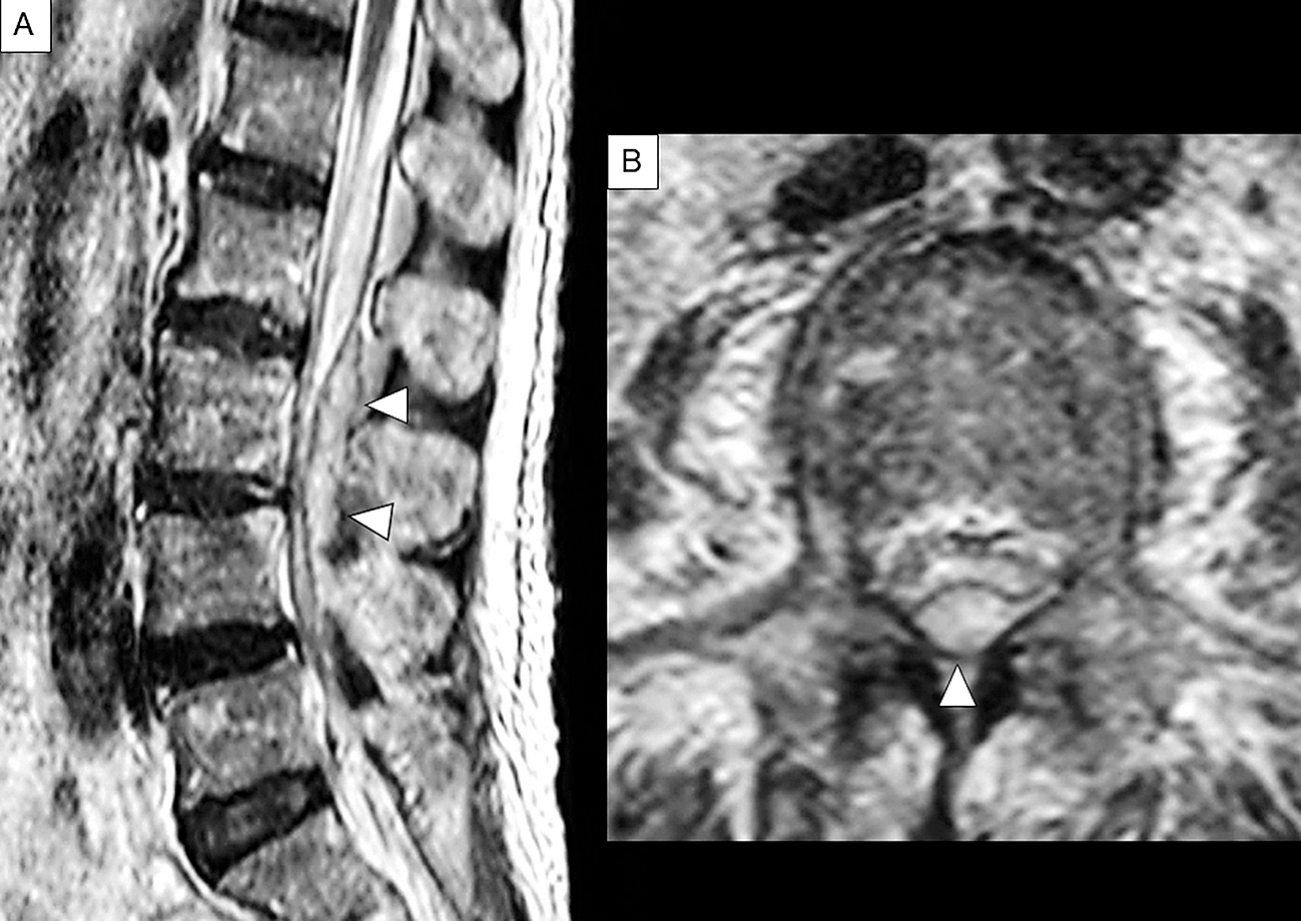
Sagittal and axial T2-weighted MRI in case 1. Extensive epidural phlegmon is noted throughout the lumbar spine in the sagittal view (A) and in the axial view (B). Content of abscess shows heterogeneous high intensity, accompanied by low intensity of capsule-like rim, which indicate granulation tissue (A). The axial view confirms that the dural tube is compressed posteriorly at the level of L3 (B). Arrowheads indicate SEA.
